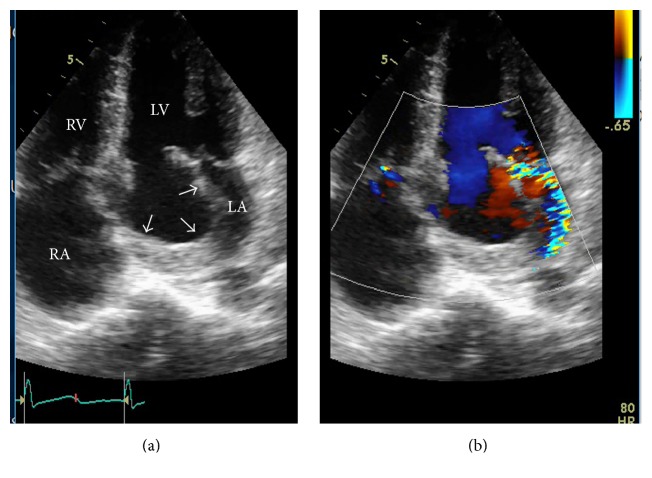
Apical 4-chamber view showing the mass (arrows) mimicking anterior leaflet aneurysm. Color Doppler (b) shows systolic flow into the mass in addition to eccentric MR. LV: left ventricle, LA: left atrium, RV: right ventricle, RA: right atrium, and MR: mitral regurgitation.
